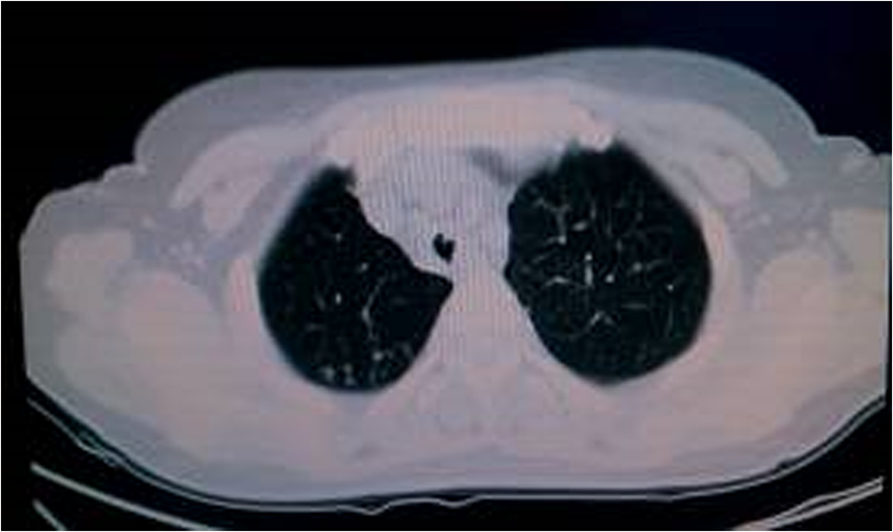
A chest computed tomography scan showing tracheal stenosis and right bronchial stenosis of the patient in Case 2
